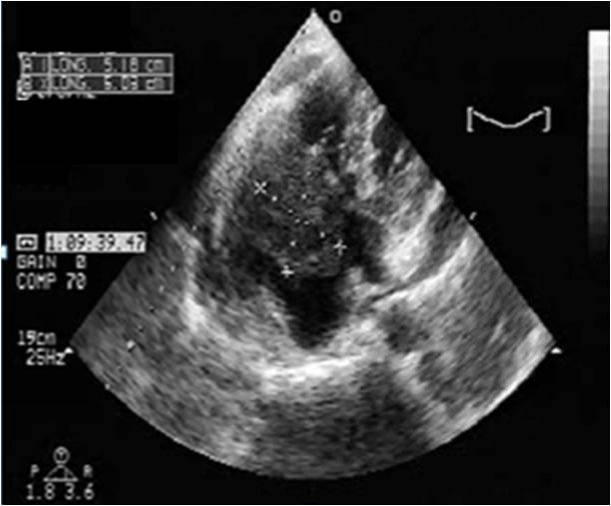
Transthoracic echocardiography four chamber view showing a pericardial effusion and a large mass. The mass measured 5.5 cm× 5 cm in the right ventricle and was attached to the tricuspid valve creating a tricuspid stenosis. The tumor has spread over the right atrium.
